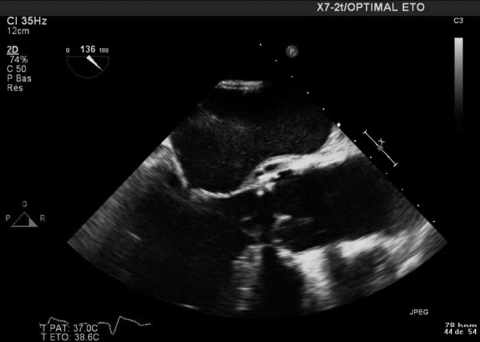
Transesophageal echocardiograph of patient with Legionnaires’ disease, Marseille, France, July 2008.
